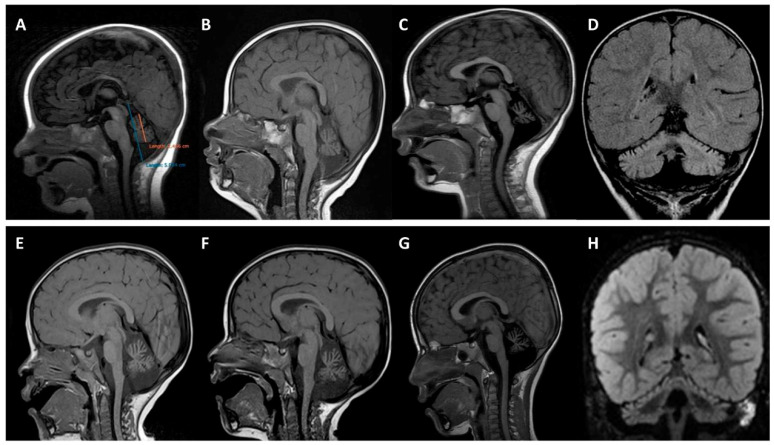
Brain magnetic resonance imaging. Upper panels (A–D) correspond to patient 1 (MD-219; p.K65E) and bottom panels (E–H) to patient 2 (MD-207; p.D255G). (A) Quantitative analysis using midsagittal vermis relative diameter (MVRD). The ratio (vermis diameter/total posterior cranial fossa diameter) was used to express the proportion of both values. The first image (A) corresponds to patient 1 at 4 months old; MVRD resulted in 54%. (B–G) Midline sagittal T1 demonstrating cerebellar vermian atrophy at the age of 12 (B) and 19 months (C) with an MVRD of 48% and 43% respectively, in patient 1; and at the age of 22 months (E), 29 months (F), and 6 years (G), with an MVRD of 59%, 57%, and 50%, respectively, in patient 2. (D,H) coronal fluid-attenuated inversion recovery (FLAIR) images exhibiting global cerebellar atrophy and predominantly superior cerebellar cortical hyperintensity (D) at 19 months old in patient 1, and (H) at 29 months old in patient 2.
